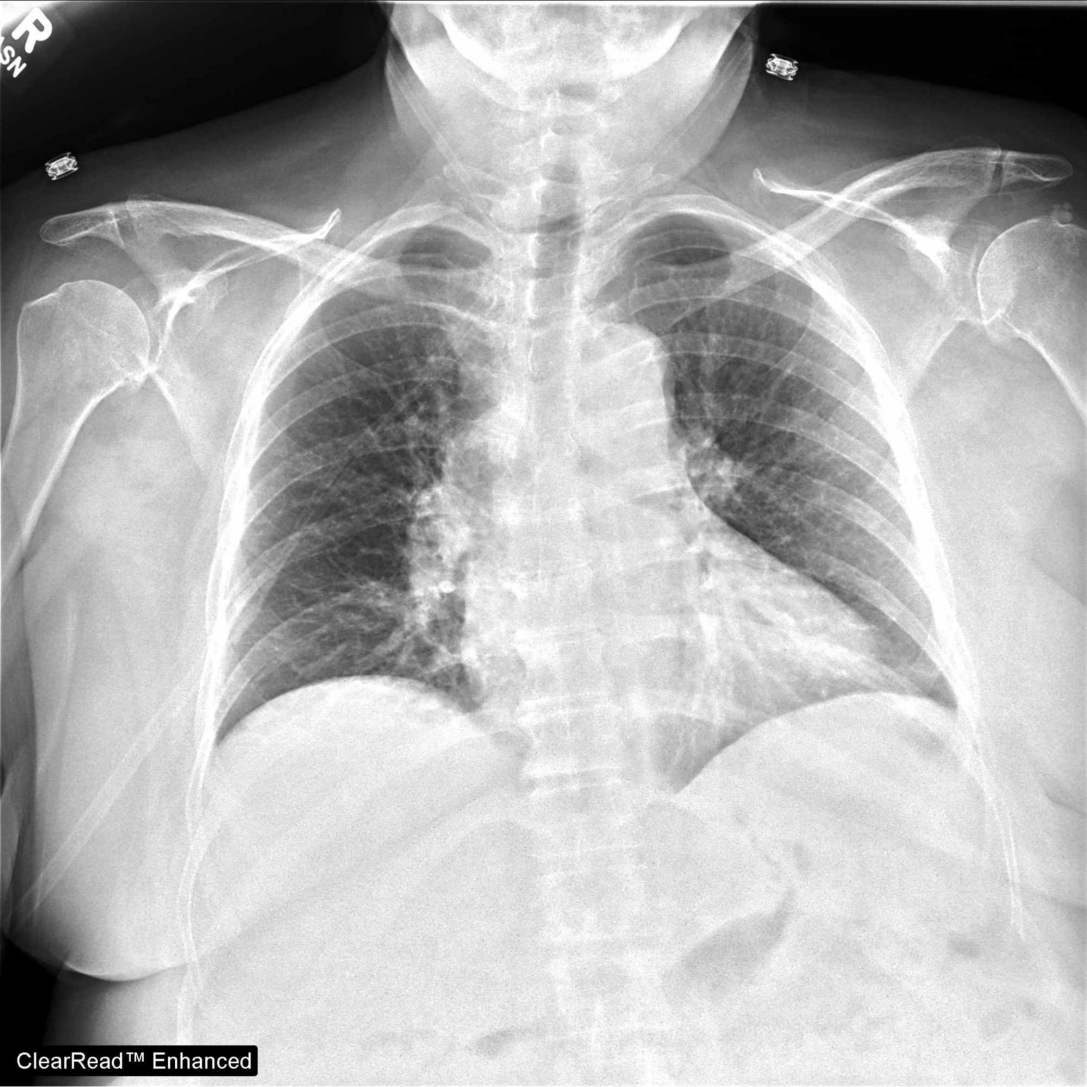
Initial Chest Radiography (CXR), with no evidence of pulmonary edema
